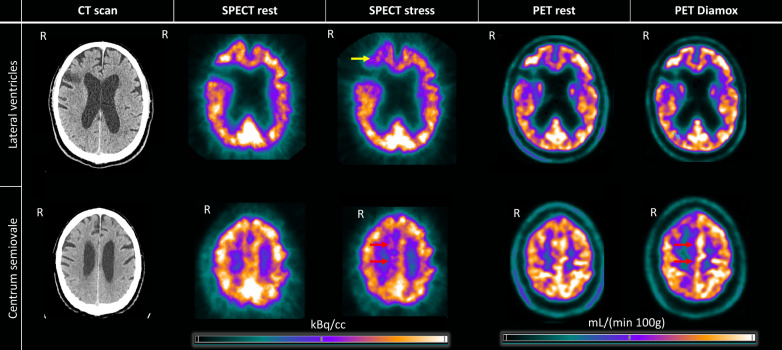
CT scan and CPI maps from the SPECT (kBq/cc) and PET (mL/(min∙100 g)) scans during rest and physiological stress or acetazolamide (Diamox®) induced vasodilation. The PET CBF maps show increased perfusion from around 40 (mL/(min∙100 g) in rest to around 70 (mL/(min∙100 g) during acetazolamide. However, the images have been visually scaled to approximate the global mean, facilitating comparison. The top row shows sections at the level of the lateral ventricles, while the bottom row shows sections at the level of the centrum semiovale. The yellow arrow indicates an area affected only on the SPECT scan, while the red arrows show affection of the mesial frontal lobe, most notably observed on the PET scan. R: right
